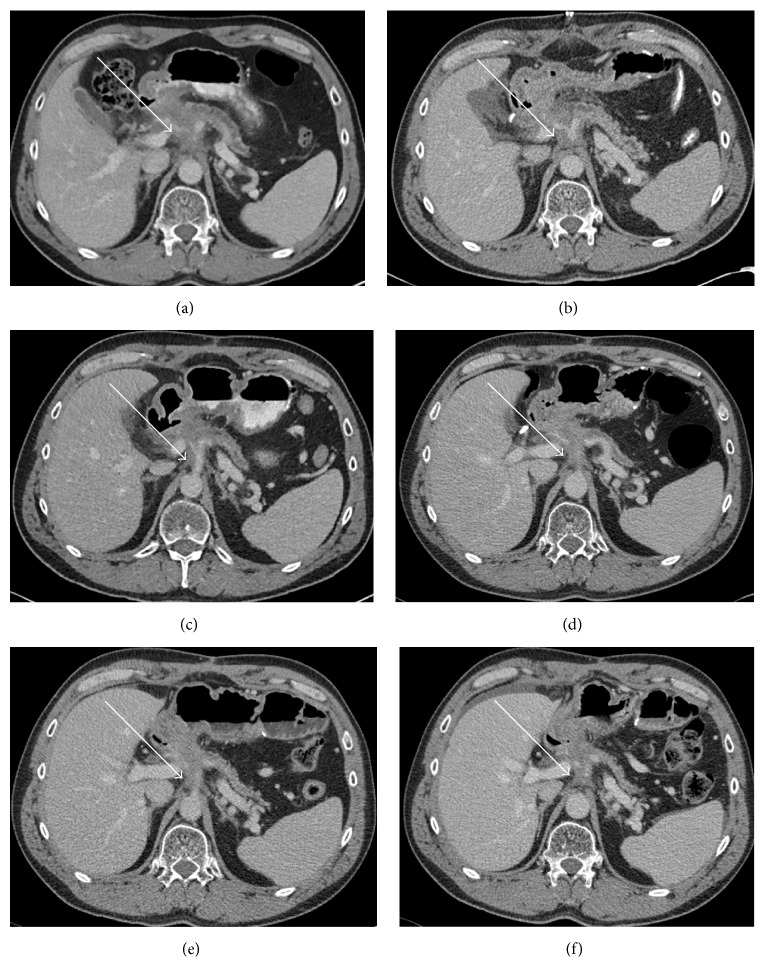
(a) Preoperative CT scan demonstrating soft tissue in pancreatic neck (white arrow) with distal pancreatic obstruction along with encasement of the celiac axis and its branches. (b) Immediate postoperative scan demonstrating irregular, hazy soft tissue with ill-defined margins in the postablative bed (white arrow). (c) Three-month follow-up scan showing similar hazy amorphous tissue in the postablation zone (white arrow) which has decreased in size. Further surveillance scans at five months (d), eight months (e), and ten months (f) demonstrating similar appearance of hazy, amorphous soft tissue which has remained stable in size (white arrows) with no signs of recurrence.
